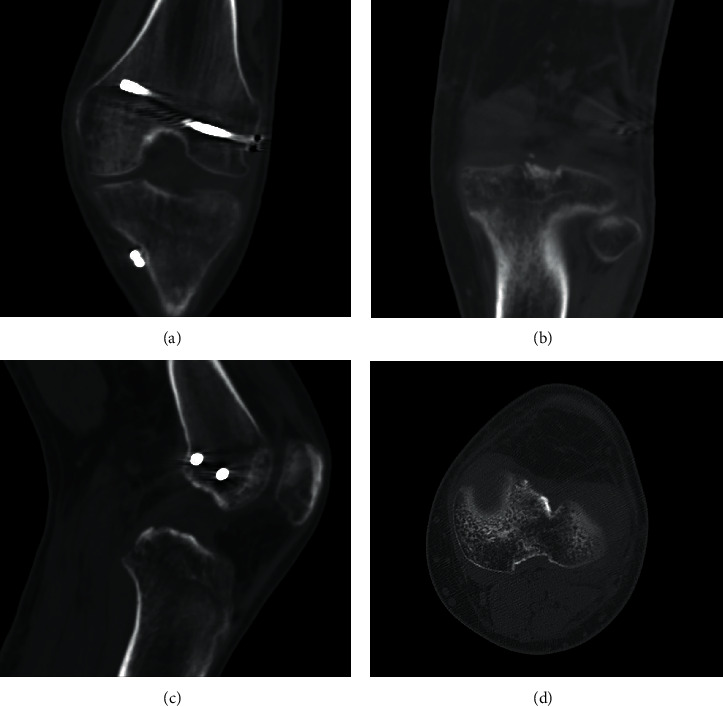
Coronal (a, b), sagittal (c), and axial (d) control CT scan after 3 months postoperative with evidence of fracture healing.
